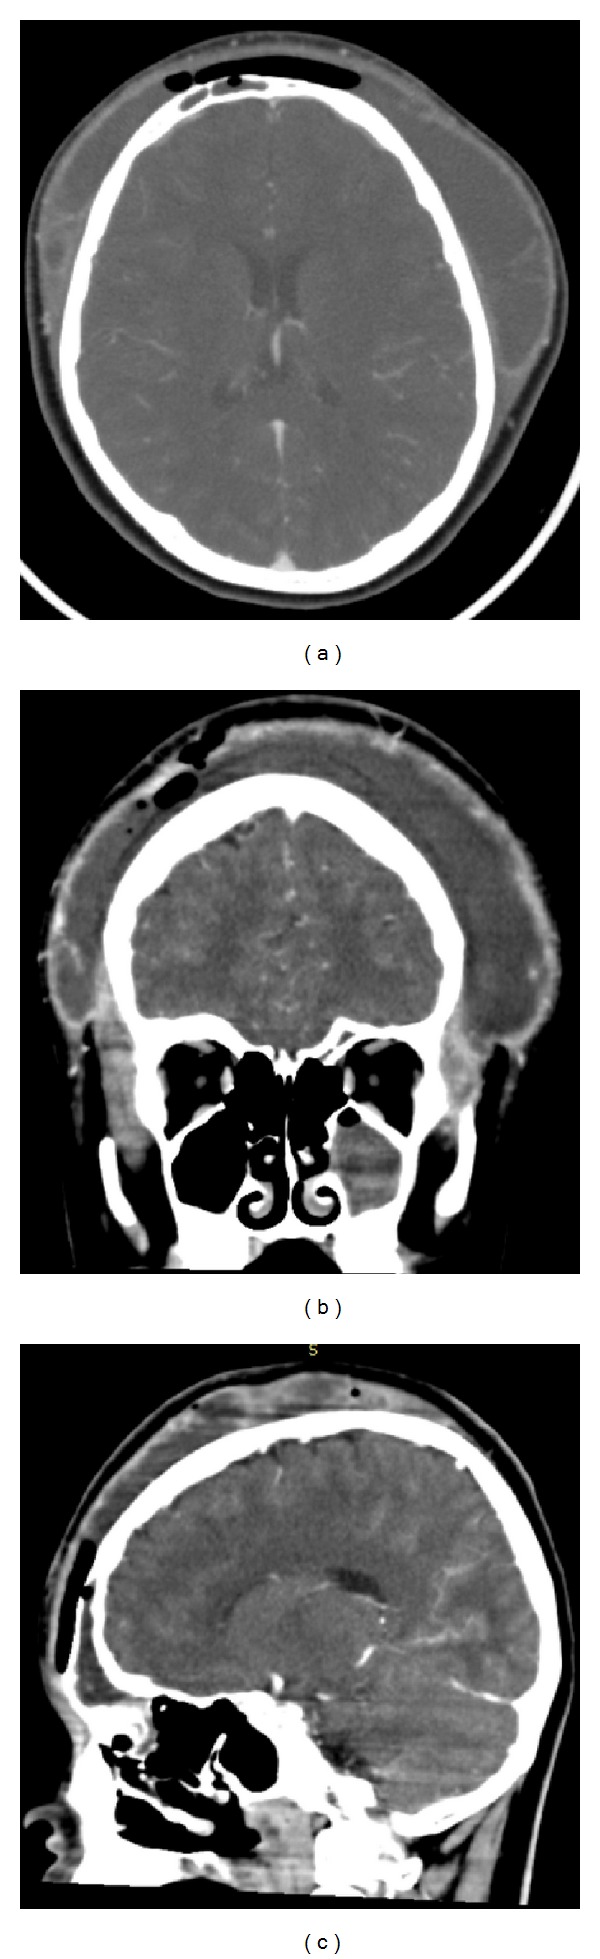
Axial (a), coronal (b), and sagittal (c) CT without contrast demonstrating soft tissue swelling of the forehead and parietal region; erosion of anterior table of the frontal sinus; frontal, maxillary, and anterior ethmoid sinuses opacification; subcutaneous emphysema.
